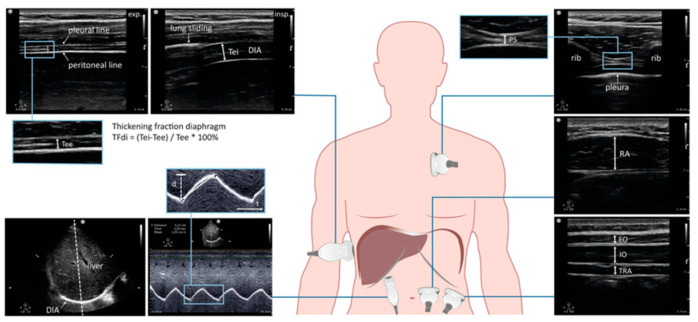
Clinical applications of respiratory muscle ultrasound (courtesy of Prof. Huiks Leo).
